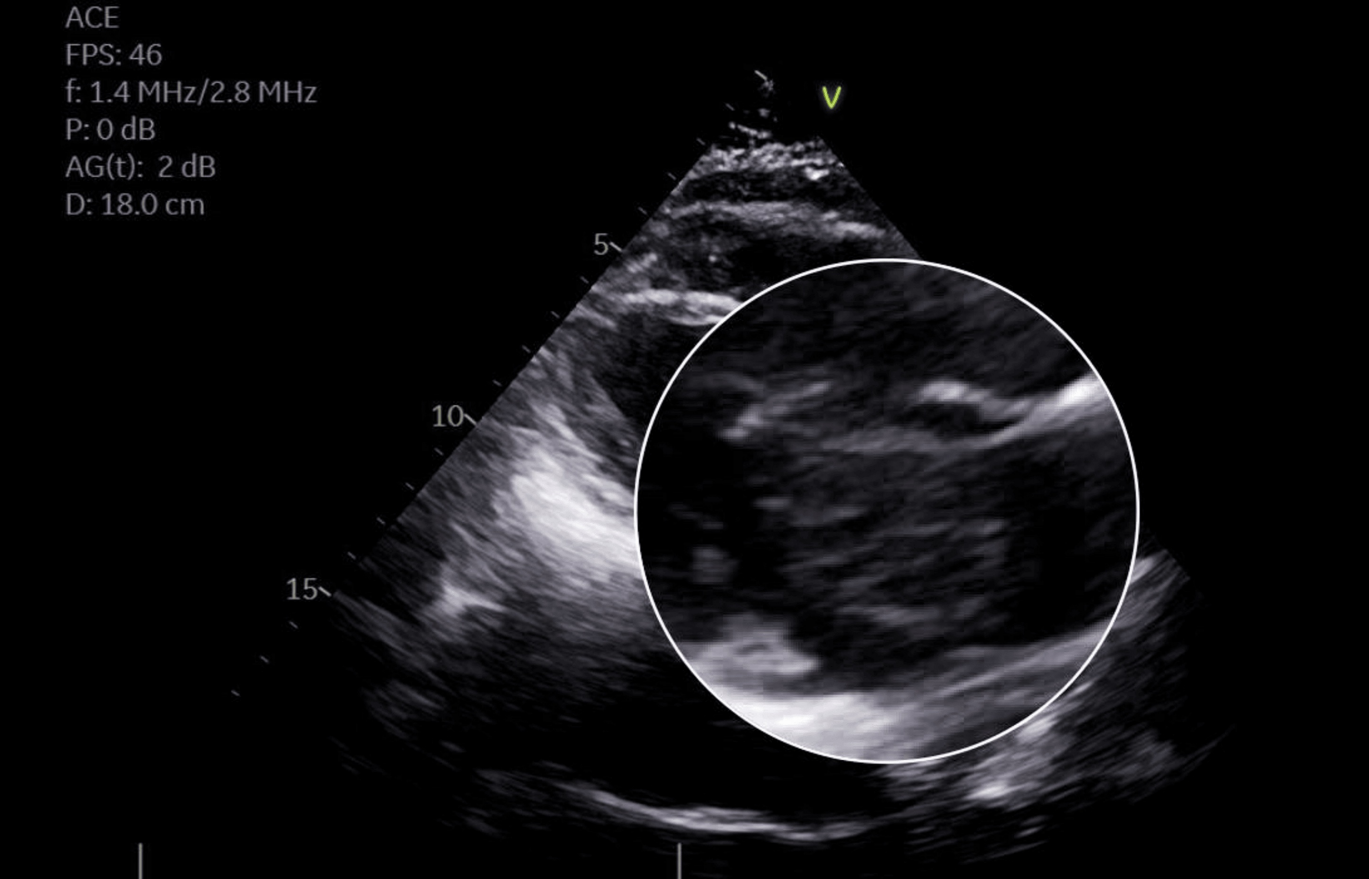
Magnified portion of echocardiogram showing a pedunculated mass in the left atrium
